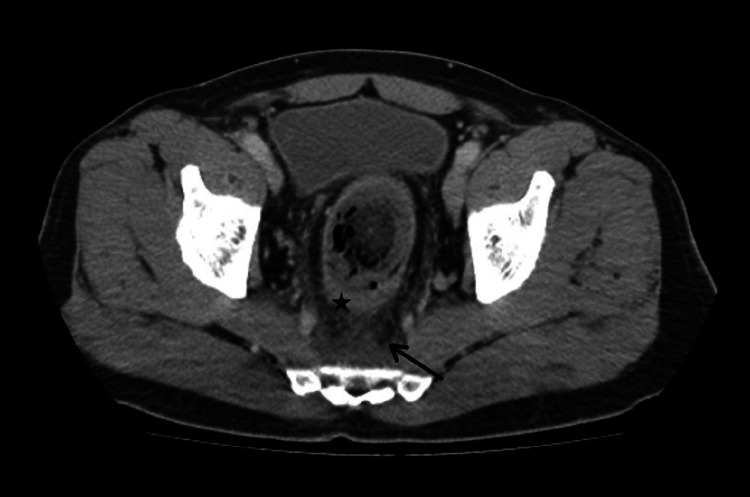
Axial CT image reveals diffuse thickening of the rectal wall (asterix), as well as edematous contamination of perirectal and presacral adipose tissue (arrow).
